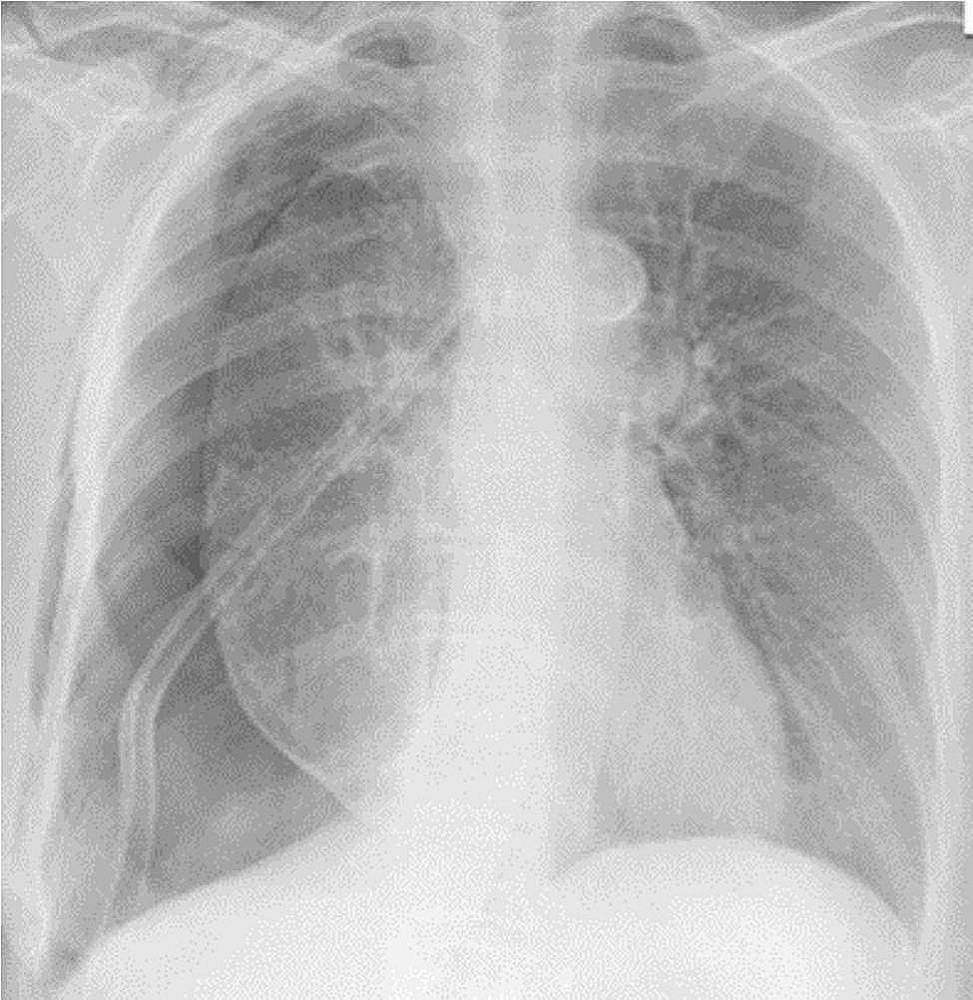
The patient’s chest x-ray before the third surgery
